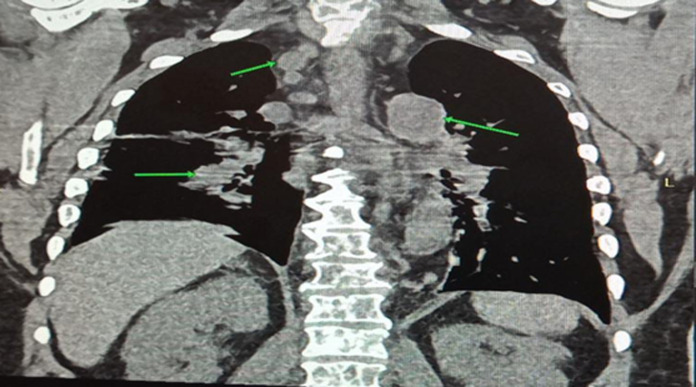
axial chest CT scan showing right para tracheal and bilateral hilar lymphadenopathy
